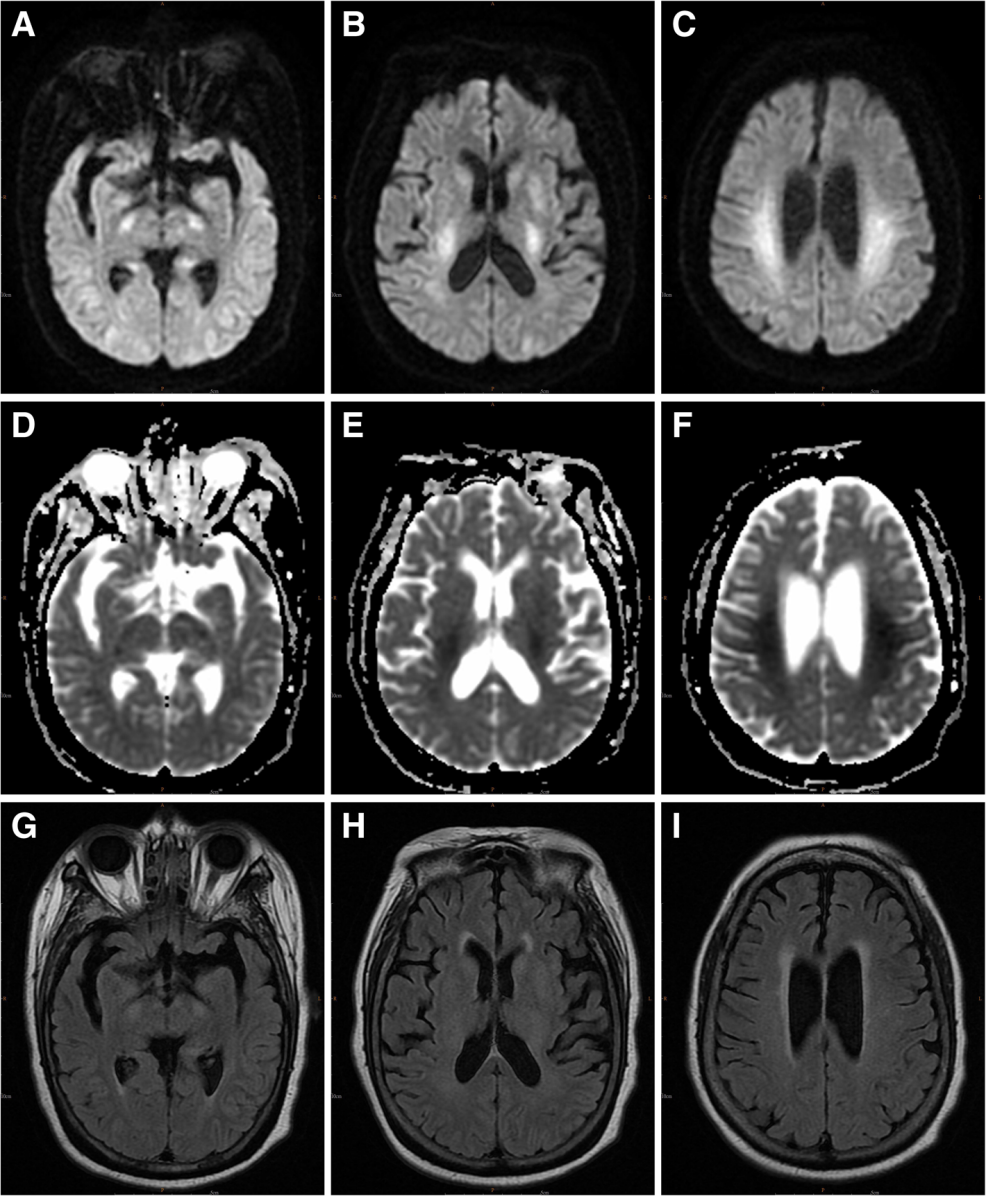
Initial axial magnetic resonance imaging conducted at the acute stage of hypoglycemic encephalopathy. Axial diffusion-weighted imaging (a, b, c), apparent diffusion coefficients map (d, e, f), and T2-FLAIR imaging (g, h, i) revealed only water diffusion restriction along the corticospinal tract, from the levels of the cerebral peduncle (a, d, g) and the posterior limbs of the internal capsule (b, e, h) to the level of the corona radiata (c, f, i), which occasionally mimic the imaging findings of acute ischemic stroke
